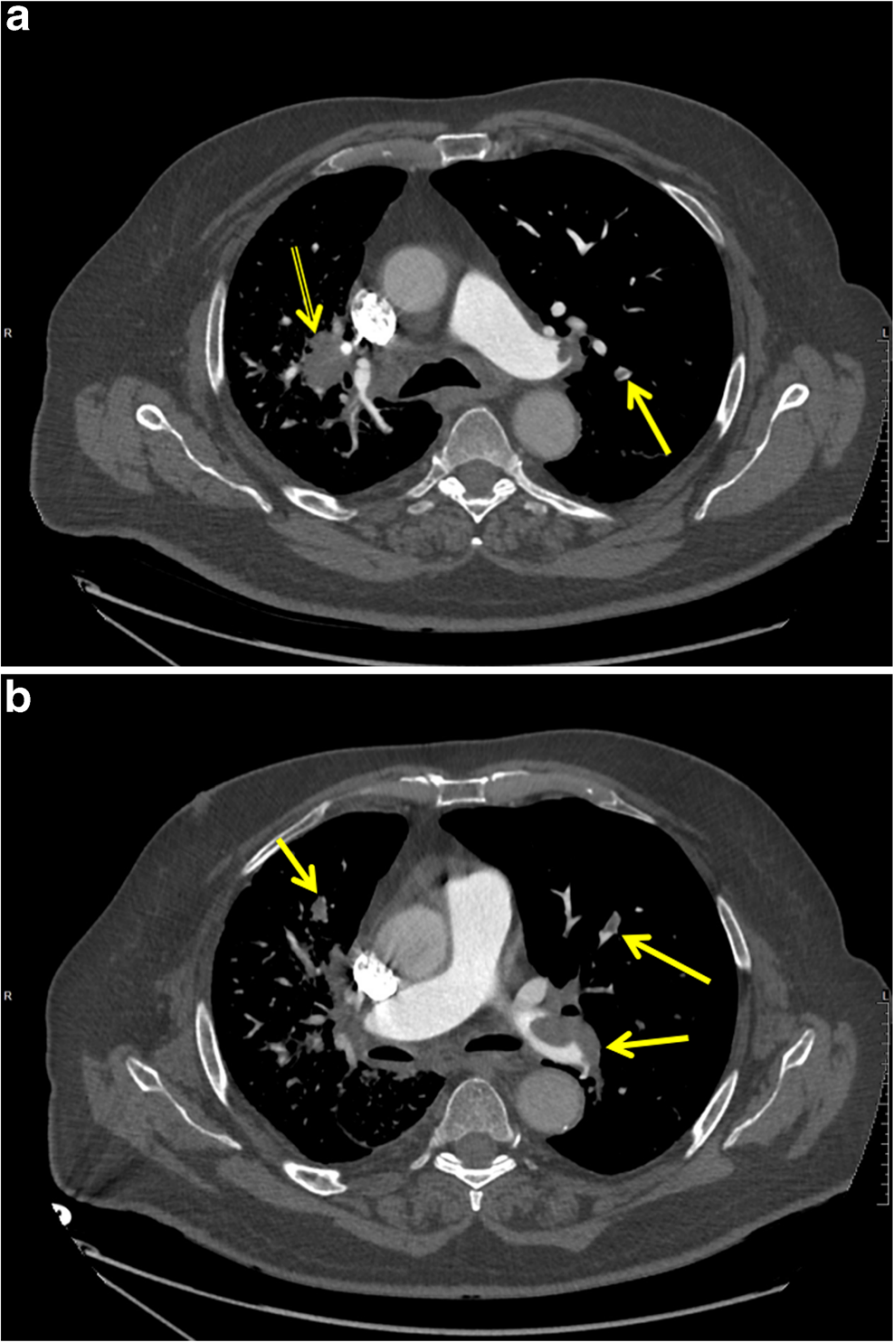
Pulmonary embolism in a 73-year-old man with known stage IV non-small cell lung cancer. Axial reconstructions of a CT pulmonary angiography study at the carinal (a) and infracarinal (b) level showing a hilar mass (*double yellow arrow*) in the right upper lobe (a) and large emboli involving the left main pulmonary arteries as well as bilateral subsegmental emboli (a, b) (*yellow arrows*)
